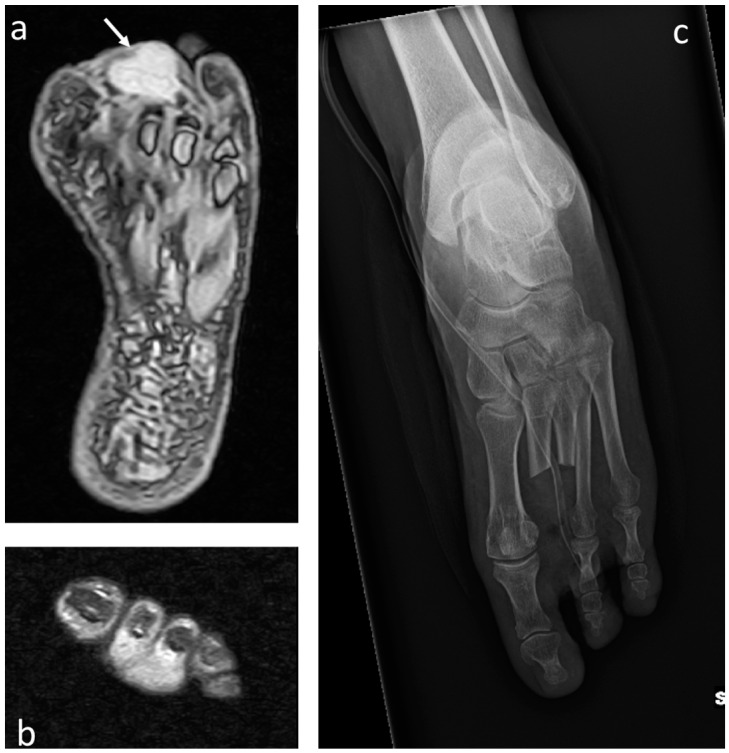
Clear-cell sarcoma of the foot (white arrows) in a 60-year-old female: (a) T2 STIR MRI showing the mass with increased signal intensity in axial and (b) coronal projection infiltrating the second and third finger. (c) Postoperative plain radiograph after double-ray amputation.
